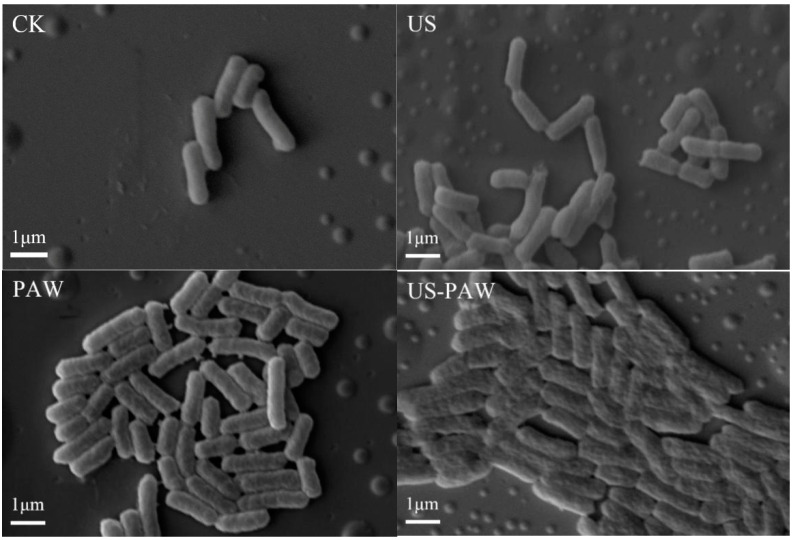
Effect of different treatments on cell morphology in *E. coli*. CK: control group. US: ultrasound. PAW: plasma-activated water. US-PAW: US combined with PAW. The scale bar is 1 µm. Original magnification: ×10,000.
